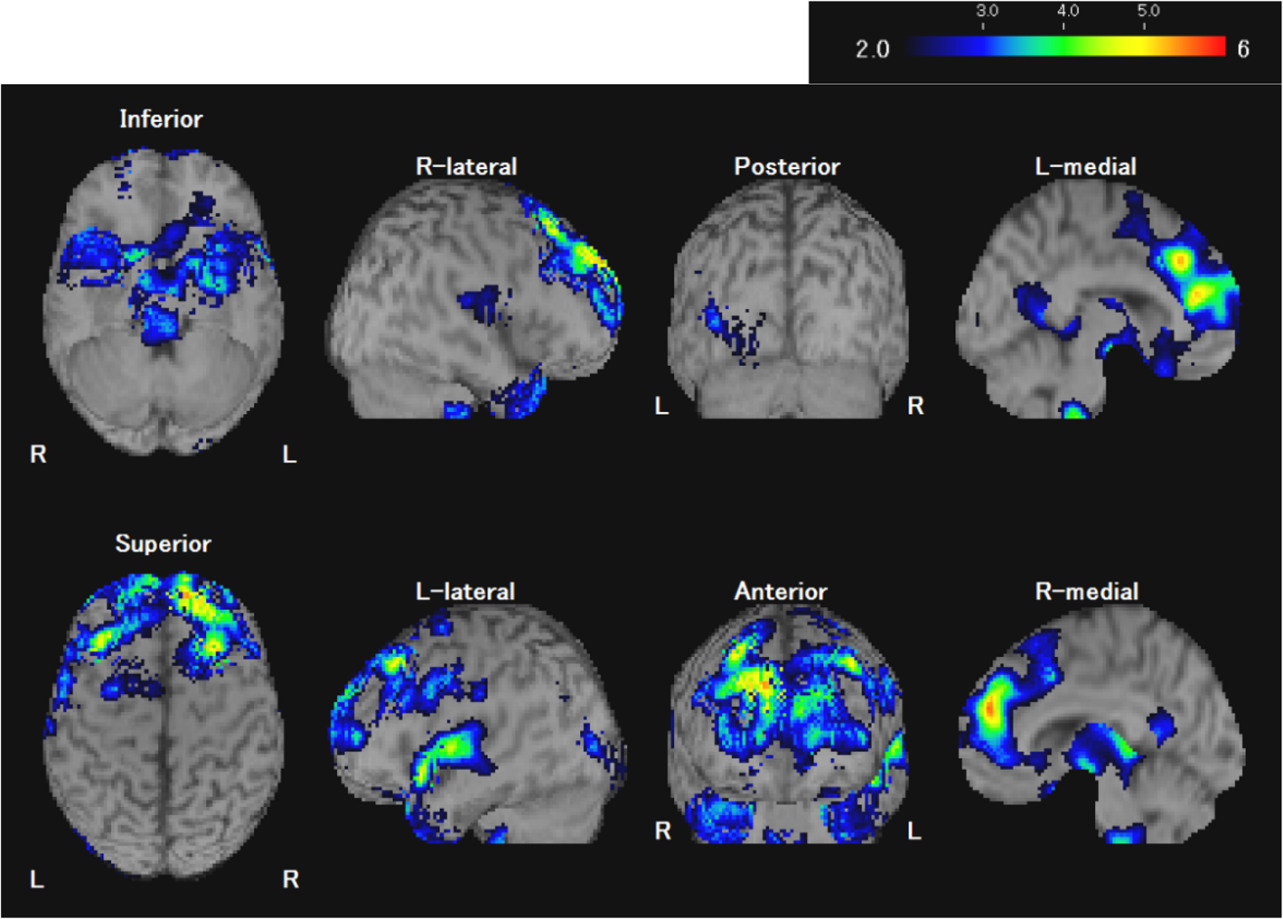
e-ZIS analysis of ^99m^Tc-ECD SPECT at 17 years of age after catatonia. The e-ZIS analysis shows decreased cerebral blood flow in the bilateral prefrontal and anterior temporal cortices
